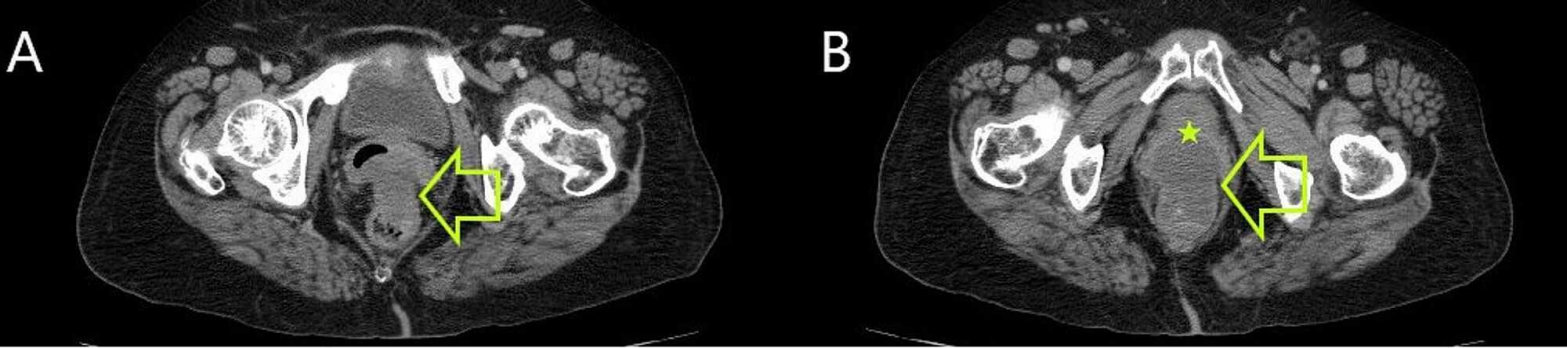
(A and B) Repeated pelvic CT showing a reduction in tumor size of 28.5% (yellow arrows) and an invasion of the posterior vaginal wall (yellow star)
